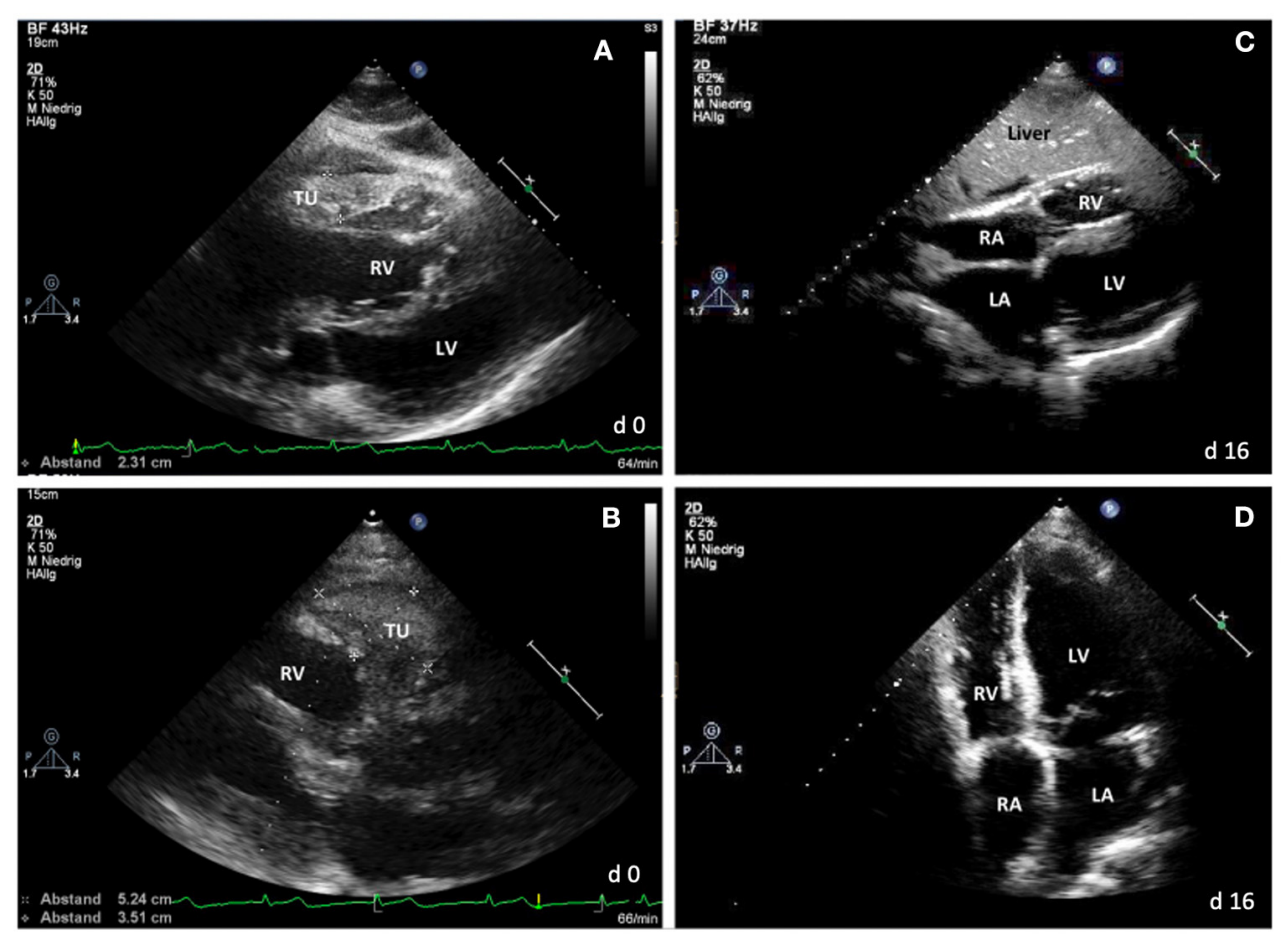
Echocardiography. (A,B) At day 0: showing a pericardial tumor and hypertrophy of the right ventricle wall. (C,D) At day 16: showing no hypertrophy or tumor mass any more. TU, tumor mass; RA, right atrium; RV, right ventricle; LA, left atrium; LV, left ventricle.
